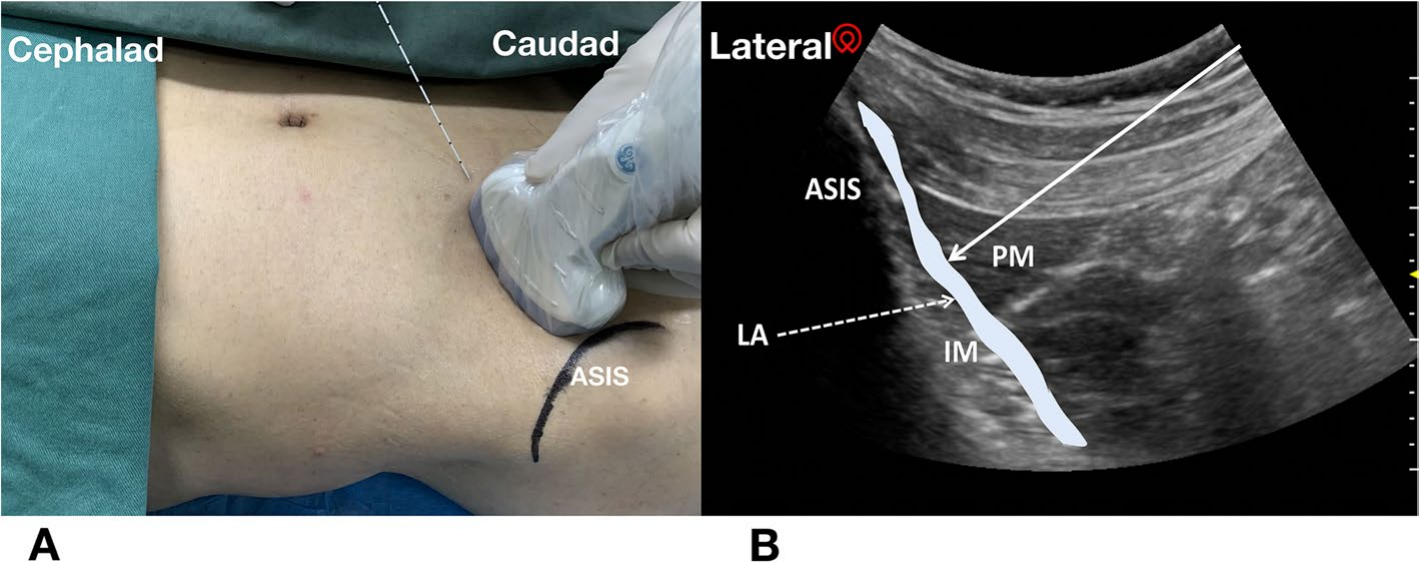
A: Posture and injection approach of fascia iliaca compartment block; B: Ultrasound image of fascia iliaca compartment block. Solid arrow indicates needle trajectory and injection point between fascia iliaca and iliac muscle (IM); dashed line indicates the spread of the LA (local anesthetic); blue:local anesthetic; ASIS, anterior superior iliac spine; PM, psoas major muscles
